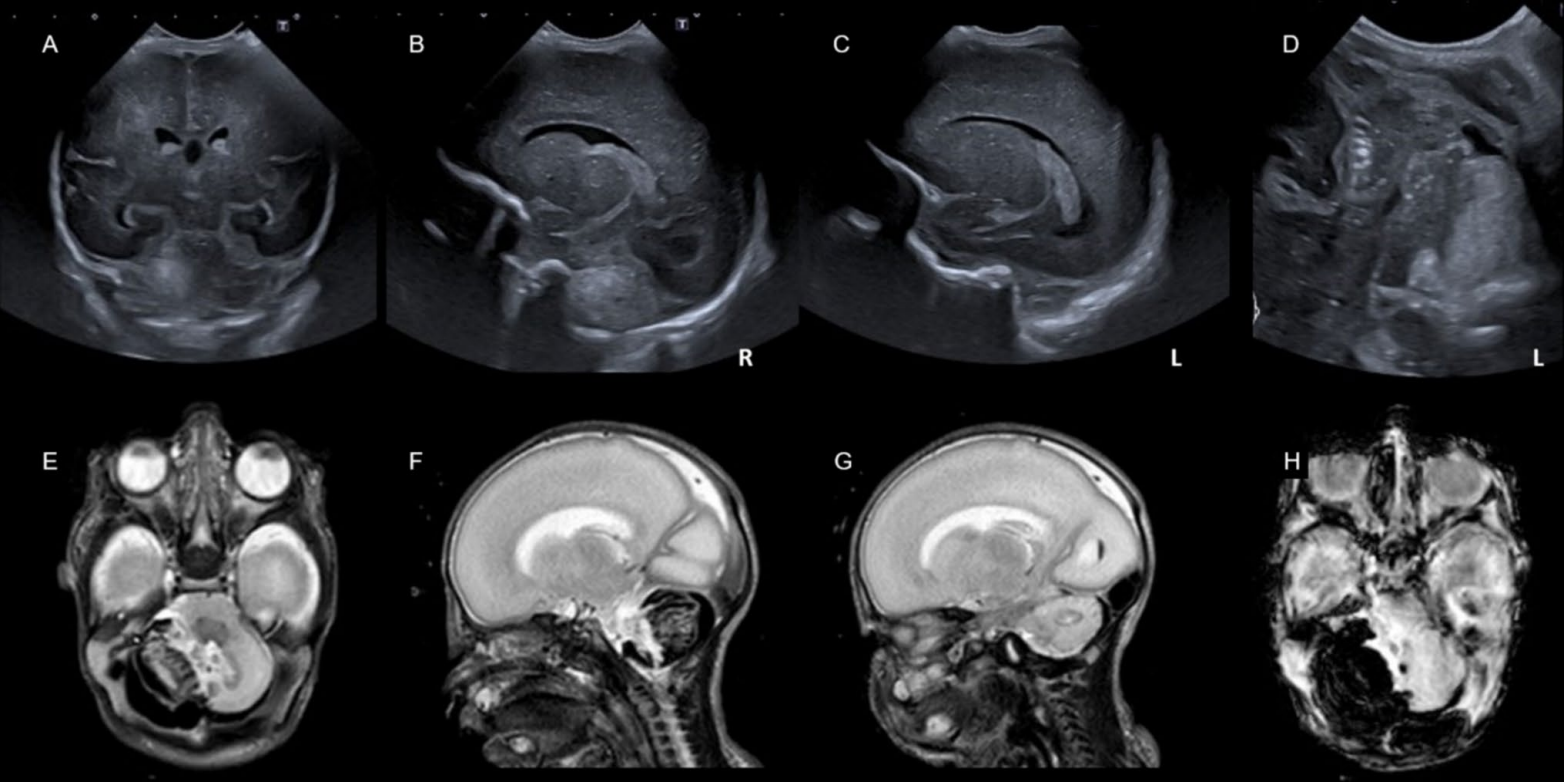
Massive cerebellar hemorrhage (CBH) of the right hemisphere in a preterm infant of 27 weeks gestational age. A Coronal scan via the anterior fontanel shows an increased echogenicity (CBH, arrow) in the right cerebellar hemisphere, which can be also seen in (B) the right paramedian sagittal scan (arrow). C the left cerebellar hemisphere appears normal (arrow). D Better visualization of the extend of the massive CBH (> 1/3 of the right hemisphere, arrowheads) via ultrasound scan through the mastoid fontanel with midline shift of the vermis and IV^th^ ventricle. E Axial T2 MRI scan and paramedian sagittal T2 scans, F corresponding to (B) and (G) to (C), and susceptibility weighted imaging (H) proving the massive CBH of the right hemisphere
